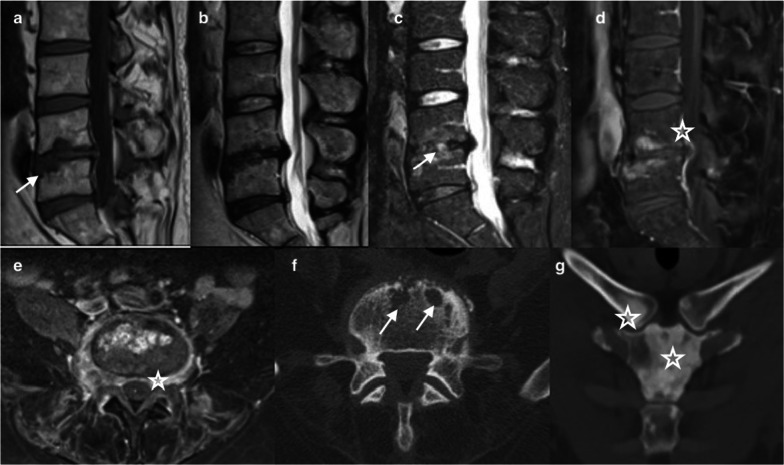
47-year-old male presenting with inflammatory back pain. Sagittal T1 (a) and R1 point 12 T2 (b)-WI show erosive L4-L5 endplates (arrow) associated on Sagittal STIR sequence (c) to a disc hypersignal (arrow) and on sagittal (d) and axial (e) T1 Fat Sat WI enhanced sequences to an enhancement of vertebral bodies and surrounding tissues as epidural fat space without abscess (star). Axial CT (f) on lumbar spine shows erosive pattern of vertebra (arrow) and Oblique reformatted CT (g) of anterior chest shows involvement with sclerotic lesions typical of SAPHO disease (star)
